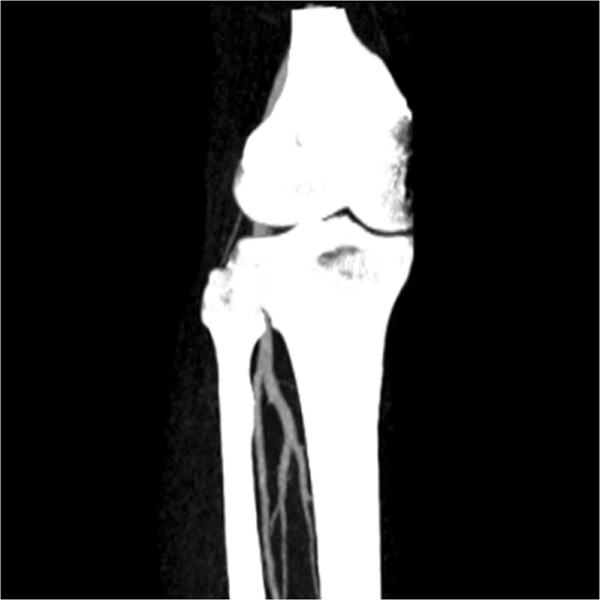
Maximum Intensity Projection (MIP) of the CTA dataset for peripheral artery.
